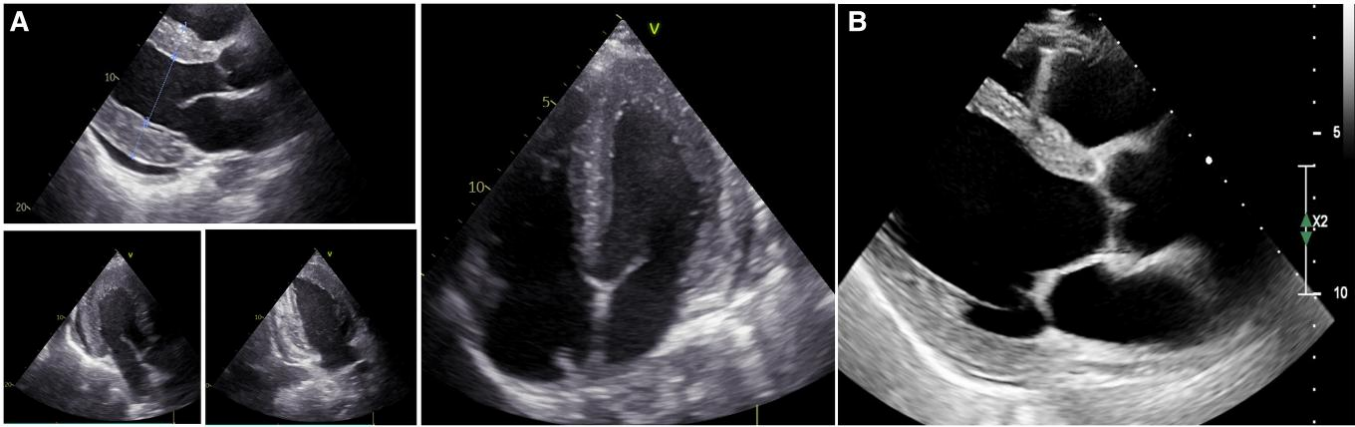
Echocardiography. (*A*) Upon arrival, transthoracic echocardiogram revealed severe concentric left ventricular hypertrophy, with an IVS wall thickness of 22 mm and a left ventricular posterior wall thickness of 20 mm, along with severely decreased biventricular function and a mild pericardial effusion. (*B*) Follow-up transthoracic echocardiogram showed a reduction in left ventricular thickness (IVS of 13 mm) and a mild improvement in left ventricular ejection fraction.
